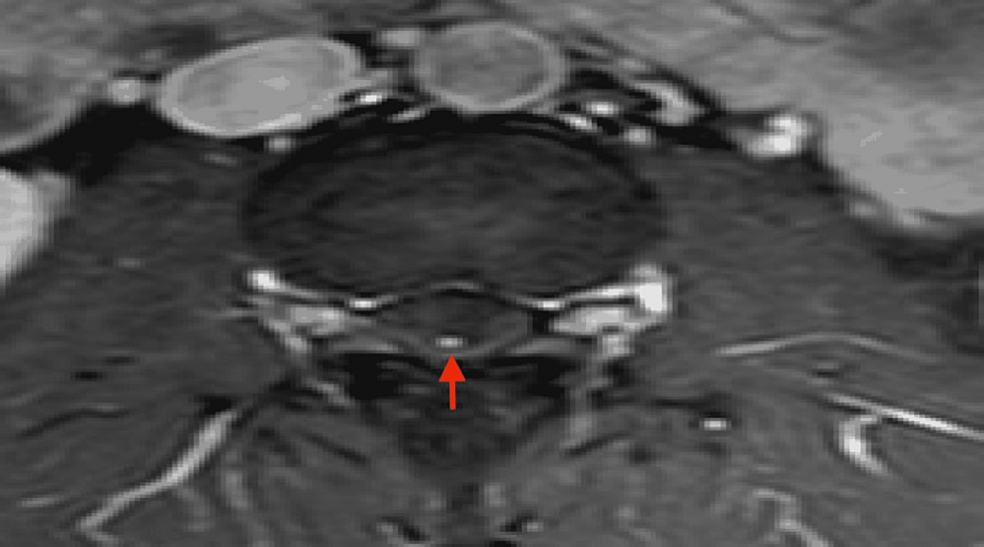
Axial T1 Gd+ MRI Axial T1 Gd+ MRI above the superior end of the tumour shows a large intrathecal vessel that continues rostrally (arrow), indicative of a hypervascular lesion.
